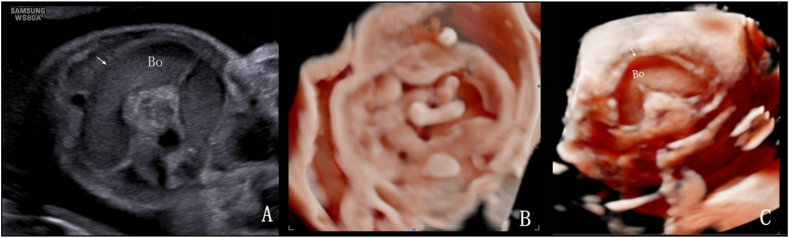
Fetus at 29 weeks and 4 days of gestation with small intestinal dilatation. Postnatal surgery confirmed terminal ileum torsion necrosis and mesenteric dysplasia. A. 2D ultrasound shows dilated intestine with the intestinal contents appearing densely packed with tiny light spots. B. In 3D imaging inversion mode, the dilated intestinal segment shows a “spiral sign”. C. 3D imaging in grayscale mode shows a smooth inner wall in the dilated intestine, indicating it is the small intestine with single-layer changes in the intestinal wall.
